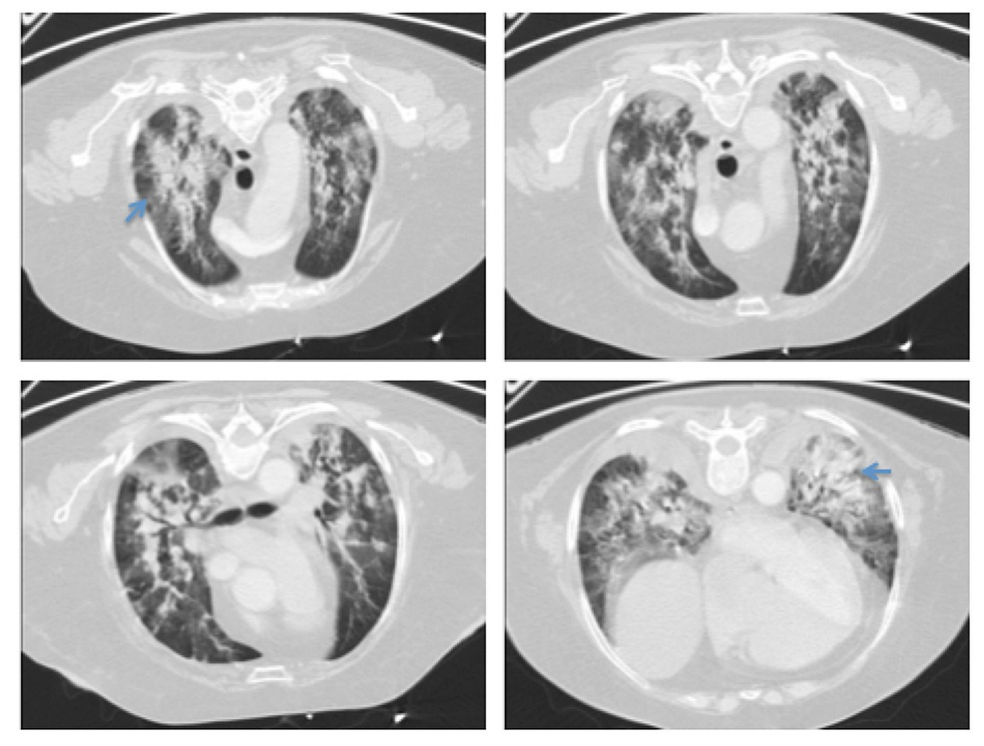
Chest CT 24 hours after admission. Chest CT scan after 24 hours of admission showed bilateral pleural effusion, with signs of alveolar consolidation centered on perihilar topography with symmetrical distribution.
